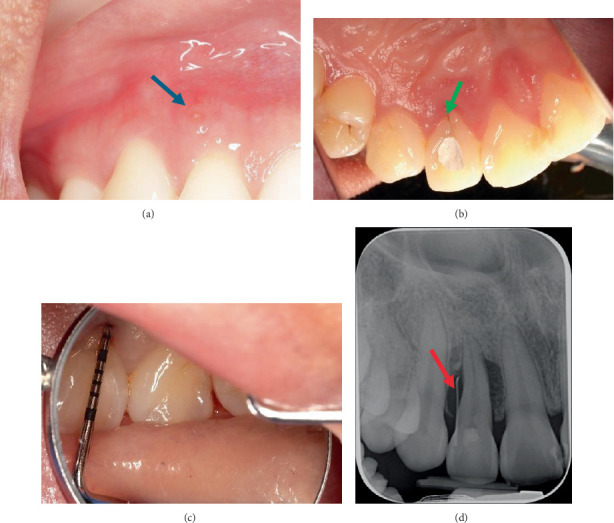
Preoperative photos of Tooth 12. (a) Buccal mucosa with draining sinus on distobuccal site (blue arrow). (b) Palatal aspect with palatal fissure (green arrow) and IRM restoration in access cavity. (c) Mid-palatal site with PPD of 7 mm and mild suppuration (purple arrow). (d) Periapical radiograph with GP point in draining sinus (red arrow).
